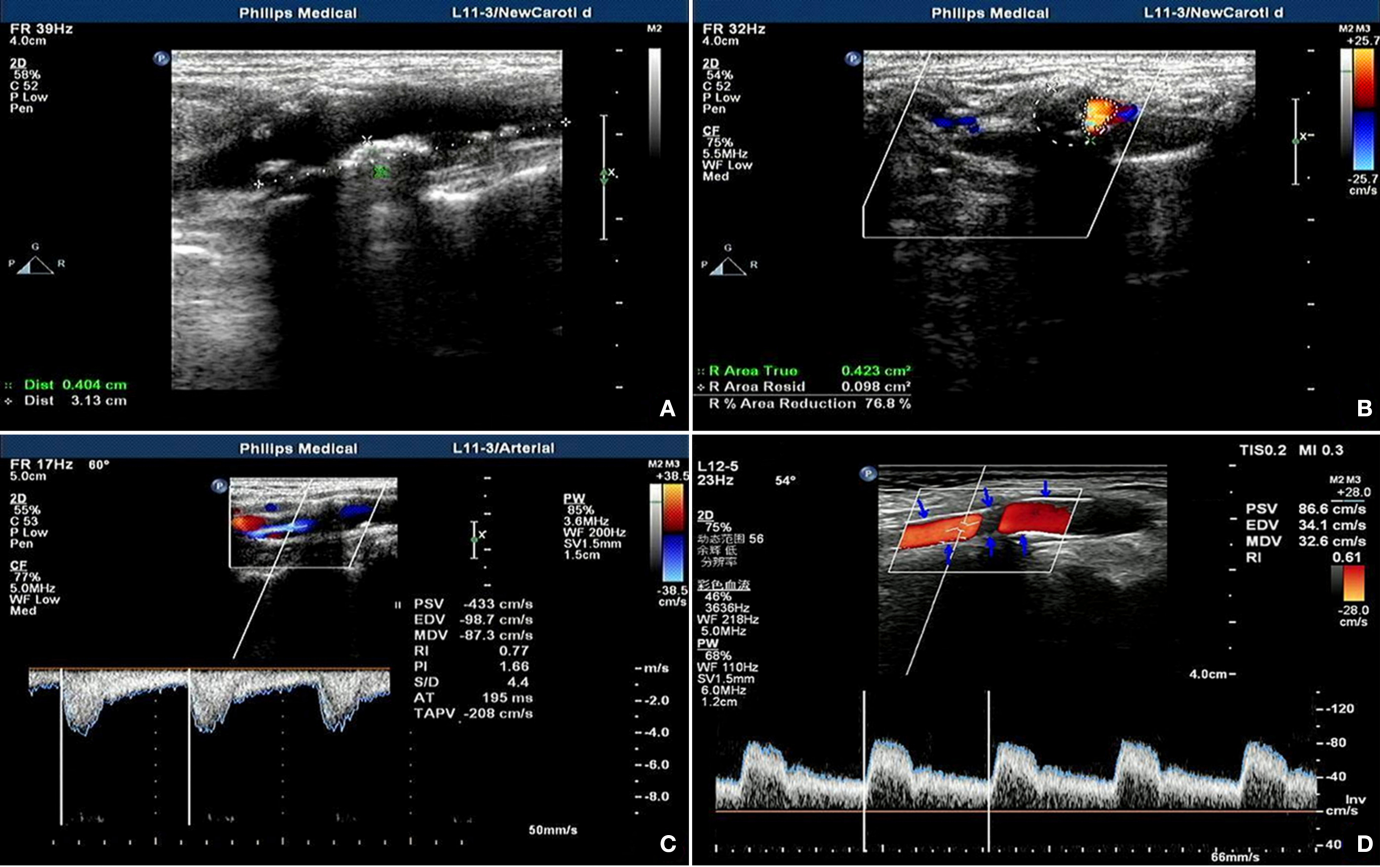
Ultrasonic manifestation before and after coronary artery stenting in patients predicted as having ideal outcomes. (A) shows multiple mixed echo mural plaques, some of which are unstable plaques; (B) shows cross section of stenosis, showing area stenosis rate of 76.8%; (C,D) show stenosis site before and after ESP respectively On the 30th day, the blood flow at the stent site showed that the shape and position of the carotid stent were good (the arrow showed the carotid stent), the blood flow in the stent was smooth, and the PV and RI were decreased.
